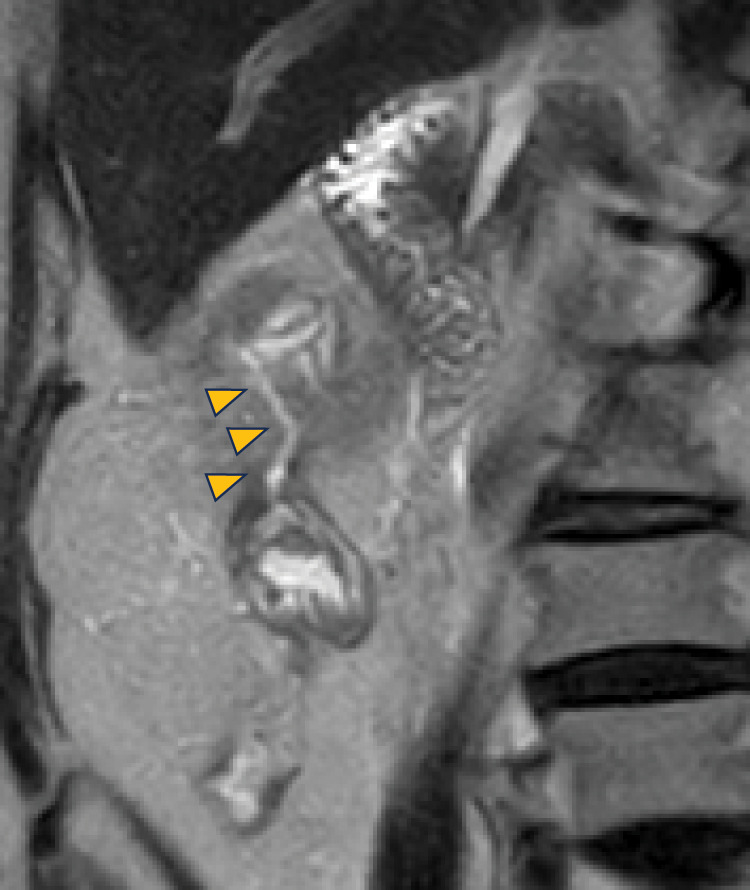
MRI findings (coronal, T2-weighted imaging). MRI performed following conservative treatment demonstrates a 35-mm mass protruding extramurally and in continuity with the anastomotic site. The cyst lumen demonstrated high T2 signal intensity without solid components. Fistulous communication between the cyst cavity and anastomotic bowel lumen is observed (arrowheads).
